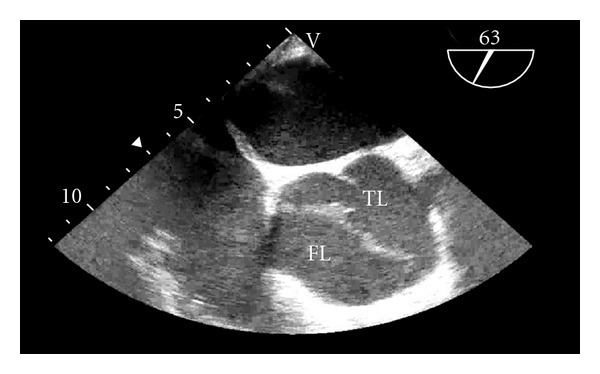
TEE image of the proximal ascending aorta (approximately at level of sinotubular junction) in mid-esophageal short axis view. The true lumen (TL) can be seen with the right, left, and noncoronary cusps. The false lumen (FL) is clearly obvious and occupies approximately forty percent of the aortic luminal area. This pathology caused severe aortic insufficiency which was demonstrable with color flow doppler (not shown).
